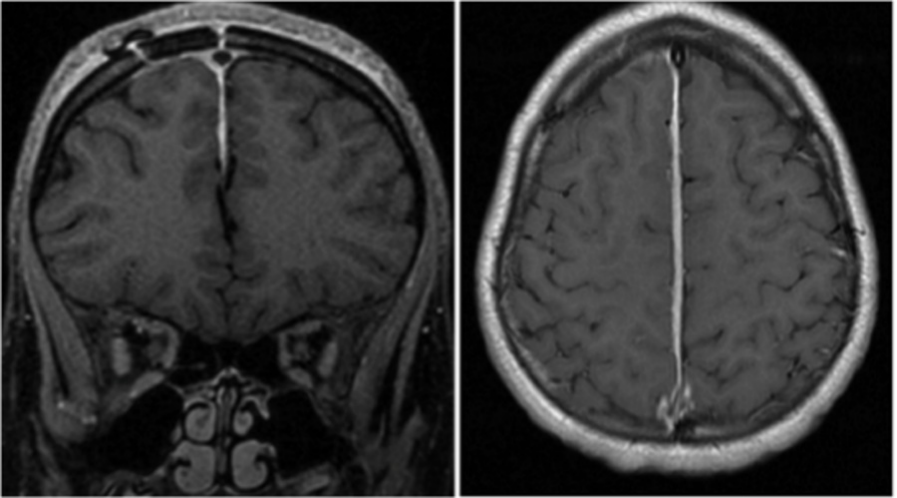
One-month post-operative (post-op) MRI showing complete resolution of prior intrafalcine empyema
